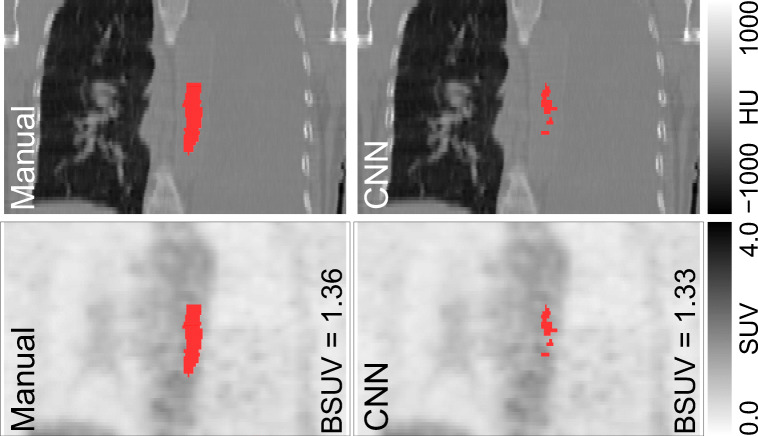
Comparison of manual and CNN-based aortic lumen delineation for a patient with pleural effusion, atelectasis of the left lung and ectasia of the ascending aorta. Coronal CT (top) and corresponding PET (bottom) images are overlaid by the respective delineations (shown in red). Note that the CNN struggles to fully identify the aortic lumen for this untypical test case, resulting in a distinctly smaller volume of the resulting ROI than is the case with manual delineation
